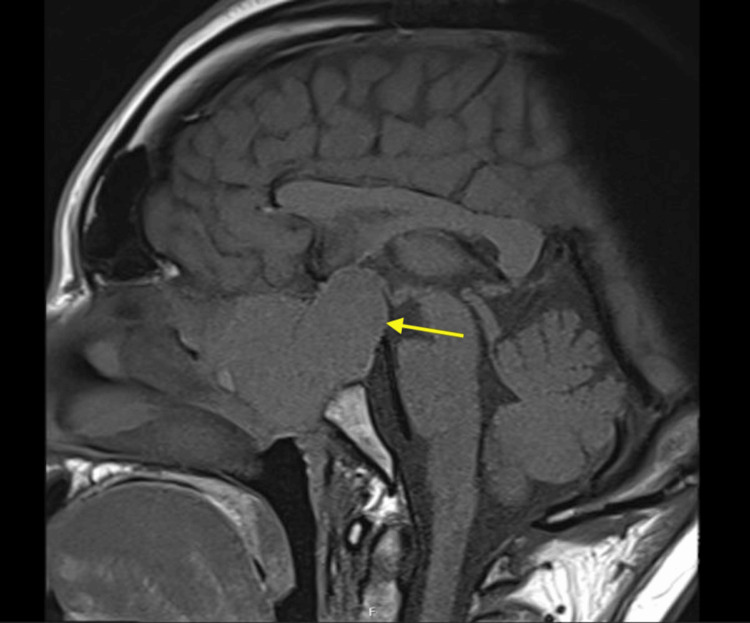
Sagittal view of the preoperative pituitary MRI Arrow pointing towards a giant pituitary adenoma eroding the planum sphenoidale, sella turcica, clinoid process and extending into the sphenoid sinus.
